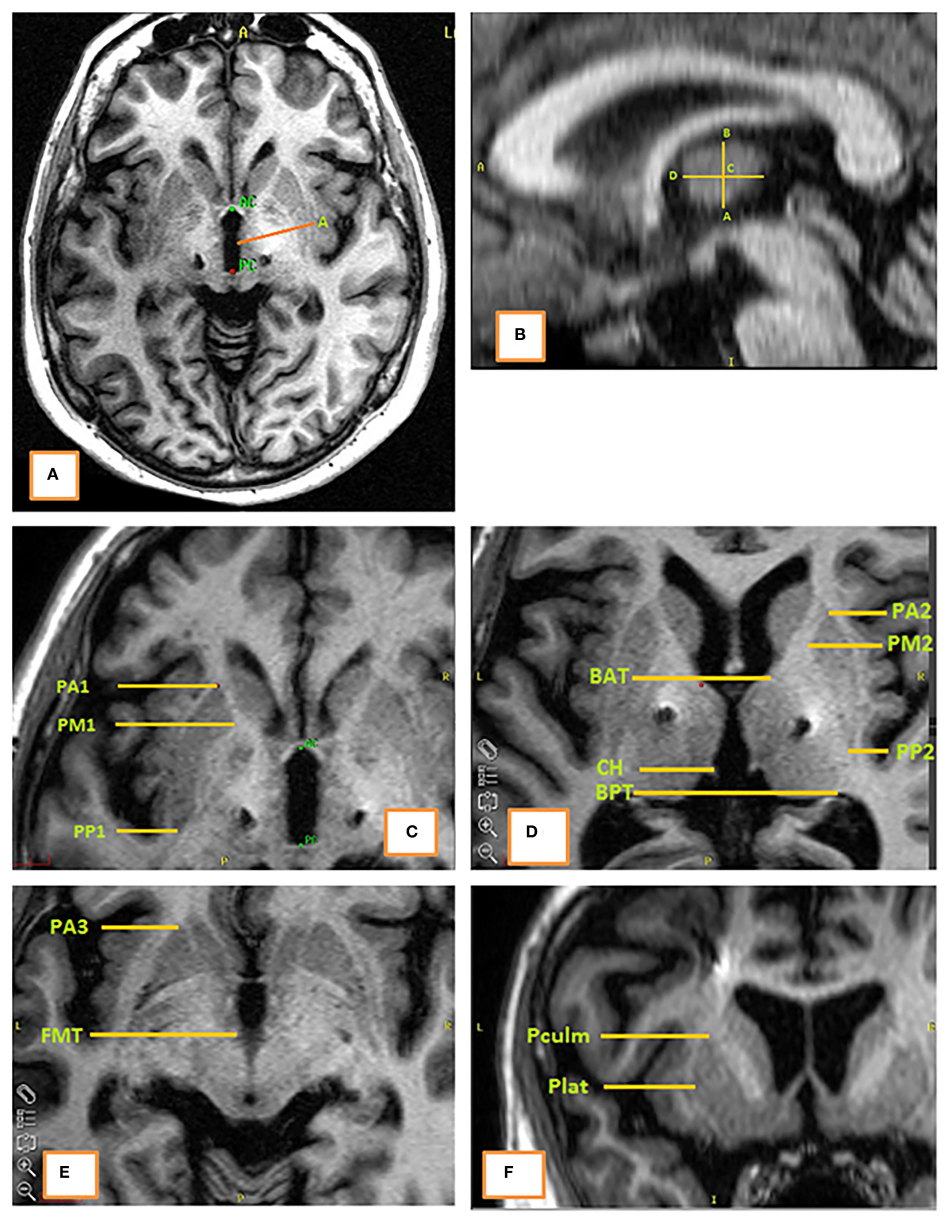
The 18 anatomical landmarks on a T1-weighted MRI. (A) Axial plane at the level of (AC-PC); (B) sagittal plane at the level of landmark “A” (lateral border of the third ventricle); (C) axial plane at the coordinate *z* = *Z*_C_ – 5 mm; (D) axial plane at the coordinate *z* = *Z*_C_; (E) axial plane at the coordinate *z* = *Z*_C_ – 10 mm; (F) coronal plane at the level of landmark “PM1.”
